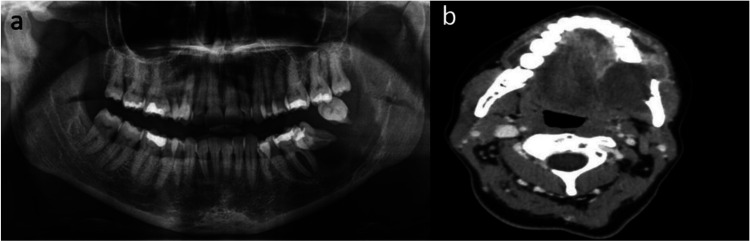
Diagnostic imaging techniques. a) OPG. b) CT scan axial view.
